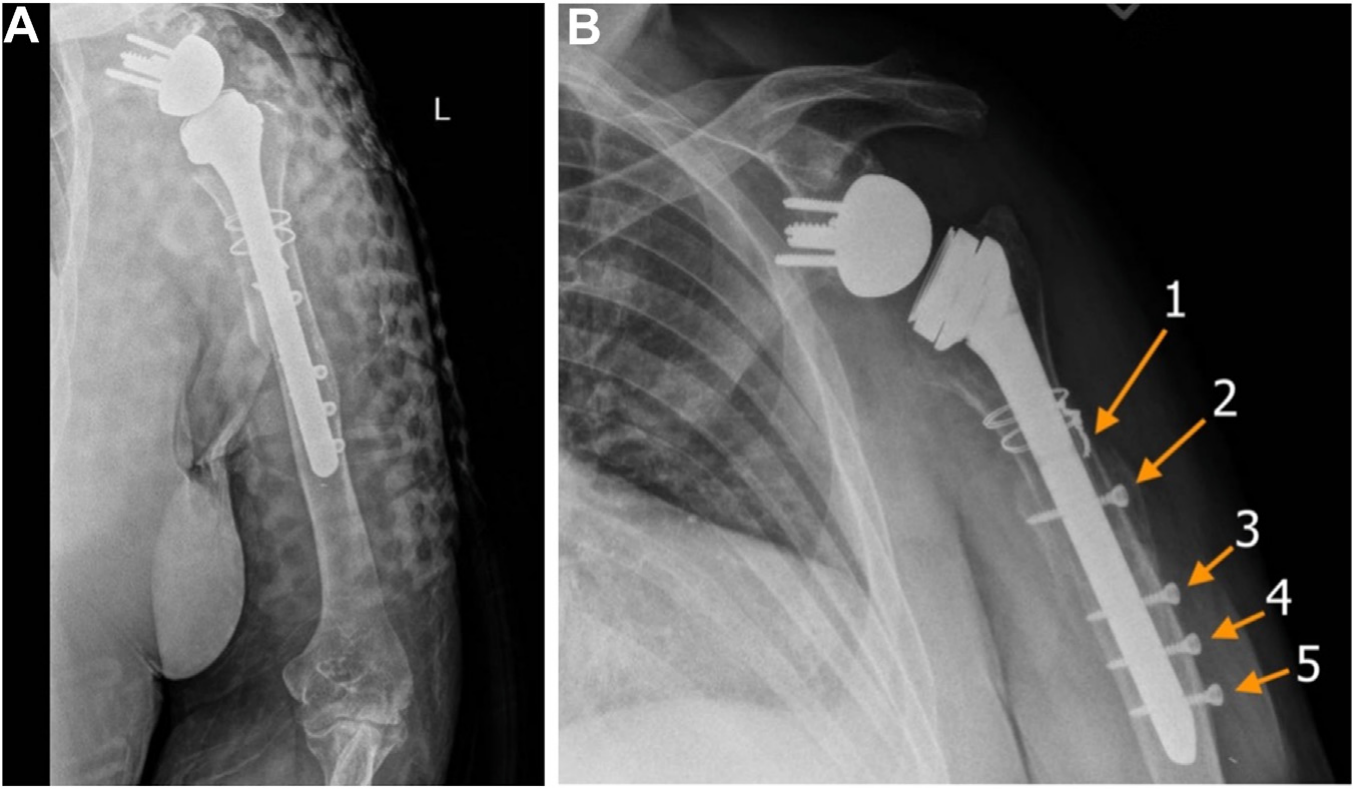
Radiographs showing a 200-mm long-stem reverse shoulder arthroplasty prosthesis with 4 interlocking screws. (A) Anteroposterior view taken immediately after reverse shoulder arthroplasty for fracture. (B) Grashey view taken 4 months postoperatively, showing interval healing. Screw hole 1 and screws 2-5 are labeled.
